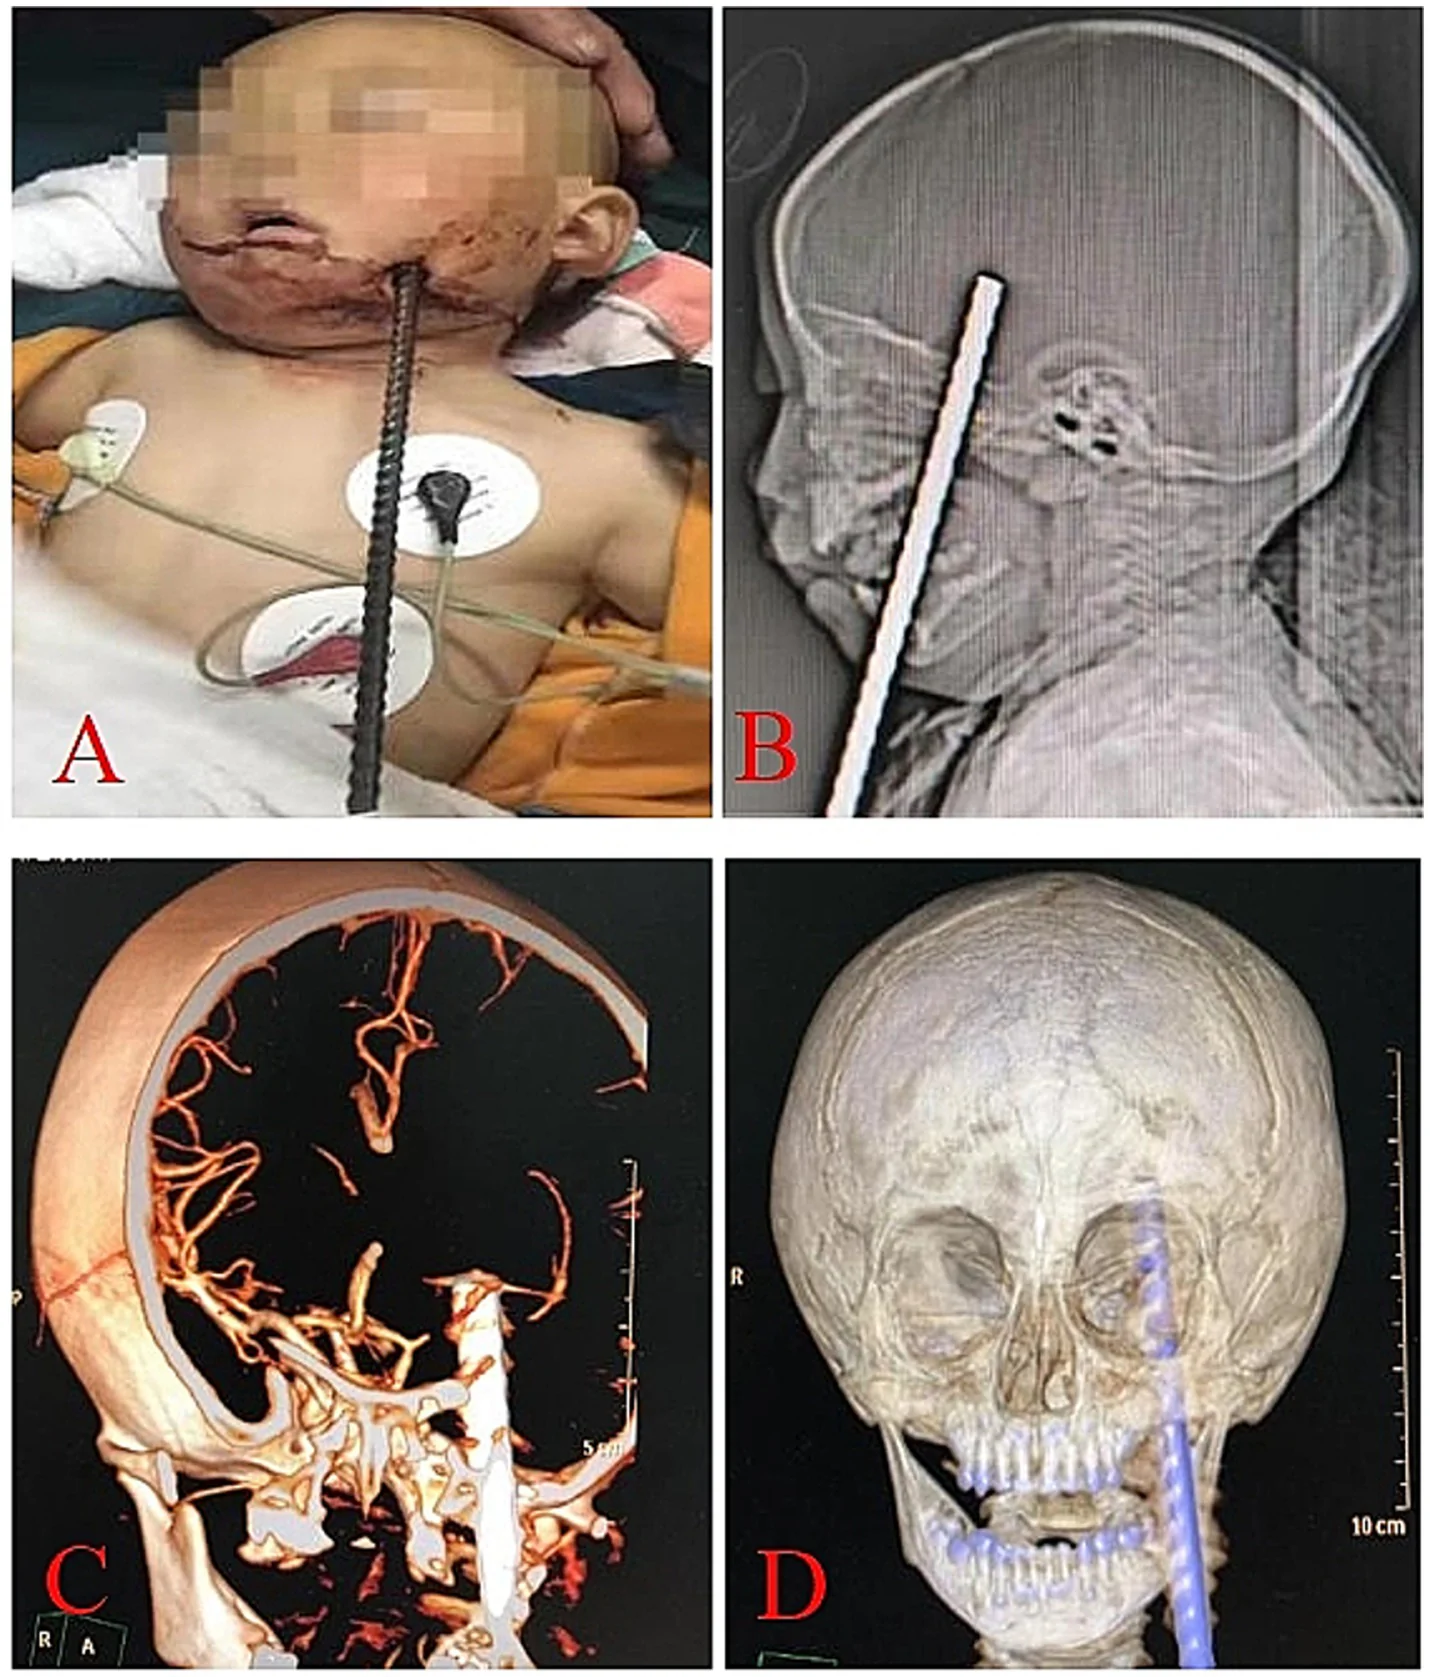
(A) Rebar penetrating the left upper jaw bone. (B) X-rays plain radiography demonstrating that the rebar penetrated about 5 cm into the skull. (C) Computed tomography angiography demonstrating left middle cerebral artery which was compressed by rebar. (D) Computed tomography reconstruction showing a hyperdense foreign material that penetrated from the left upper jaw bone to the deep structures in an oblique fashion.
